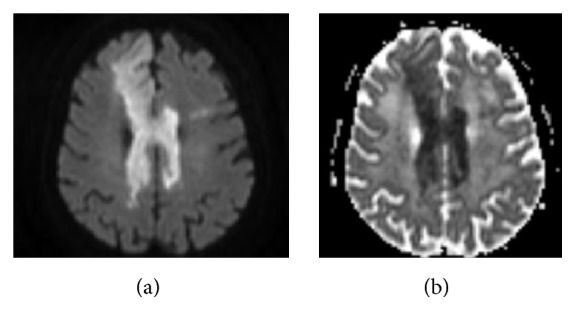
MR diffusion weighted images. DWI (a) and ADC map (b) with significant restricted diffusion of the corpus callosum bilaterally in all the segments and in the right anterior frontal and parietal region.
